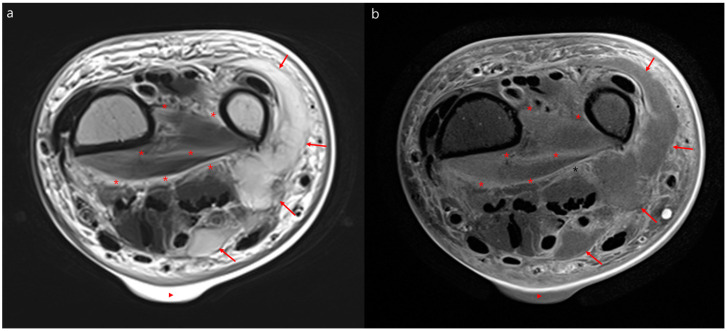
Representative magnetic resonance images of necrotizing fasciitis. Necrotizing fasciitis of the left wrist in a 71-year-old woman. Axial T2 weighted magnetic resonance image (a) and contrast-enhanced magnetic resonance image (b) showing diffuse hyperintensity with irregular enhancement of the deep peripheral fascia and intermuscular deep fascia (asterisk) of the wrist. Additionally, there is a lobulating abscess in the ulnar side of the wrist (arrows) and a skin bulla (triangle).
